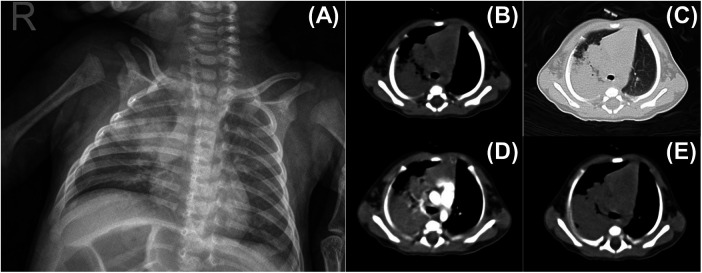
Chest radiograph and lung CT scan on admission. (A) The chest x-ray shows an abnormal opacity in the right upper lobe. (B–E) Lung CT on Day 2 demonstrates a heterogeneous mass in the right upper lobe measuring approximately 2.9 × 3.7 × 4.7 cm, with marked enhancement on contrast imaging.
